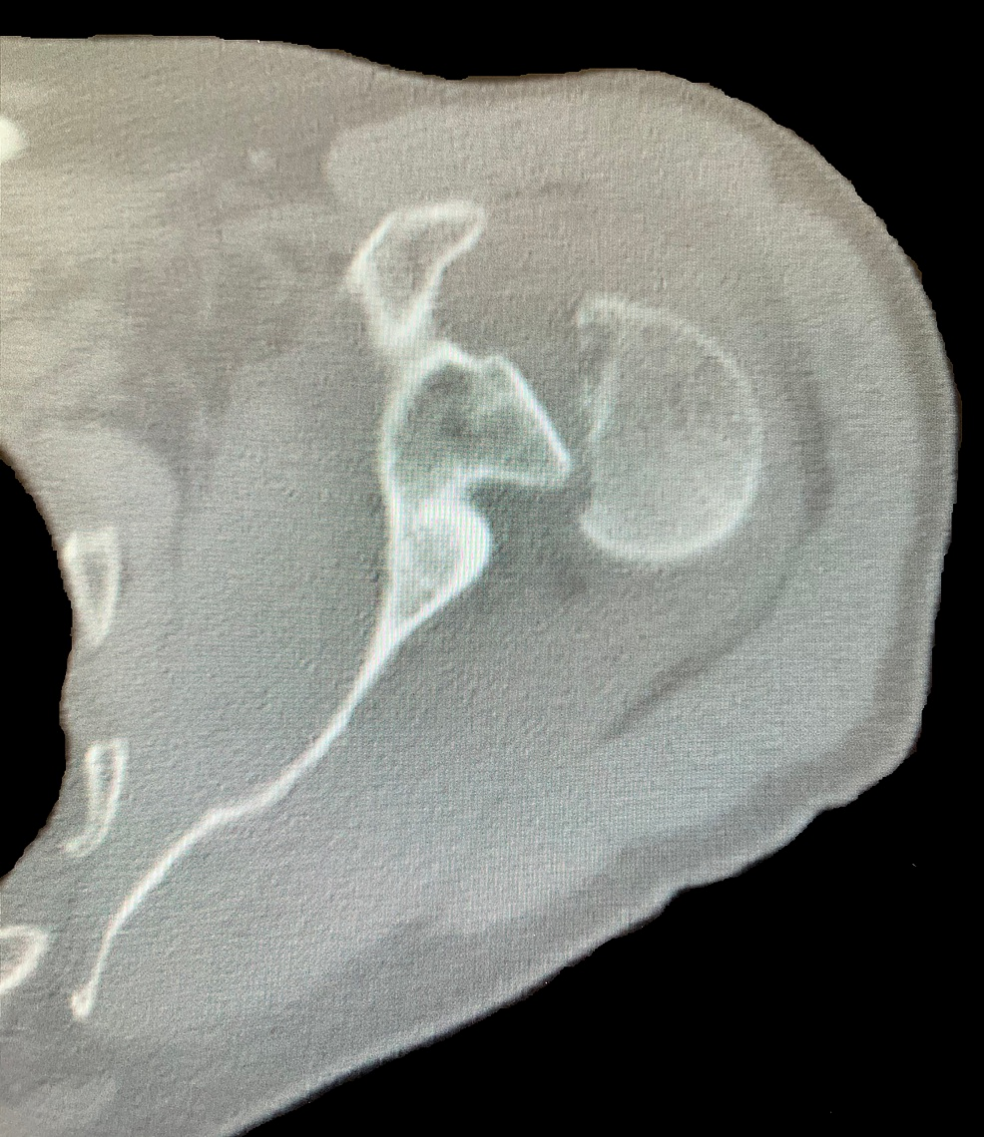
Axial view of preoperative computed tomography (CT) scan of the left shoulder demonstrating a four-part proximal humerus (PH) posterior fracture-dislocation.
